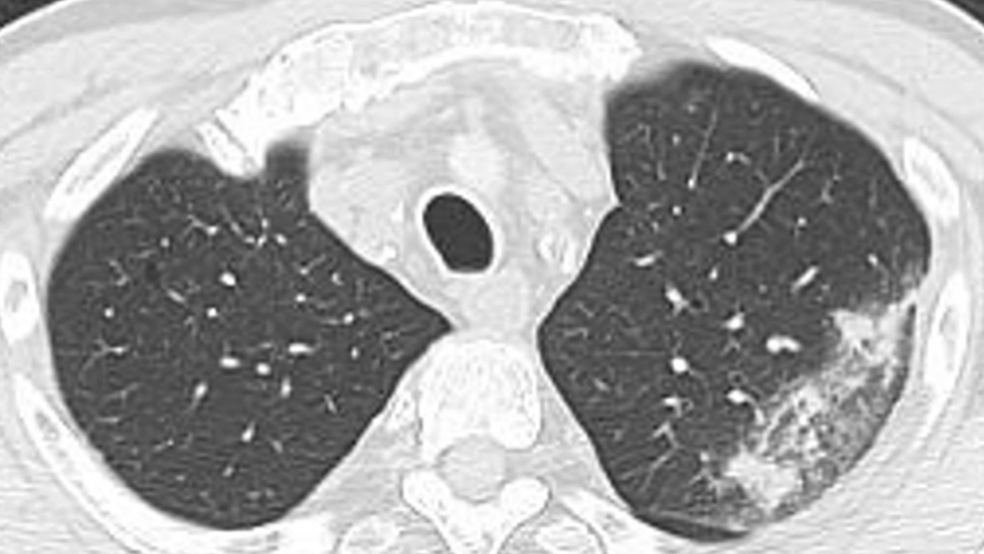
Second chest computed tomography scan revealing that opacity in the lower left lung lobe had improved but a new opacity had appeared in the left upper lung lobe.
